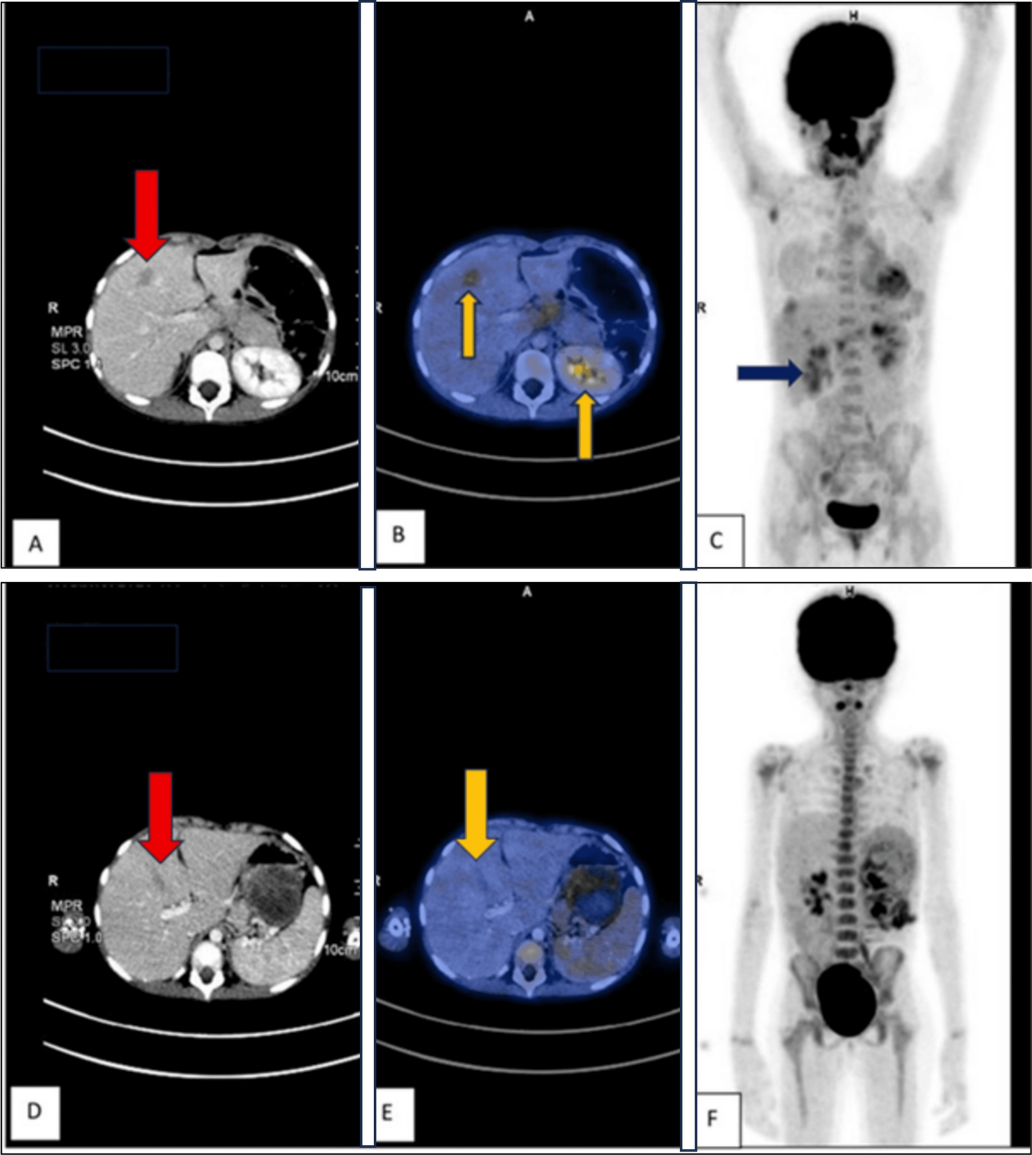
Two-year follow-up: (A) CT image showing a liver lesion with altered density (red arrow). (B) Fused PET-CT image highlighting areas of increased metabolic activity in the liver (yellow arrows), suggestive of active disease. (C) Whole-body PET image showing increased uptake in the liver region corresponding to (B) (blue arrow), indicating active disease. One-year follow-up: (D) CT image showing a smaller, less pronounced liver lesion (red arrow). (E) Fused PET-CT image demonstrating reduced metabolic activity compared with (B), suggesting less active disease. (F) Whole-body PET image showing limited or no significant metabolic activity in the liver or elsewhere, indicating a less active or earlier stage of disease PET: positron emission tomography, CT: computed tomography
